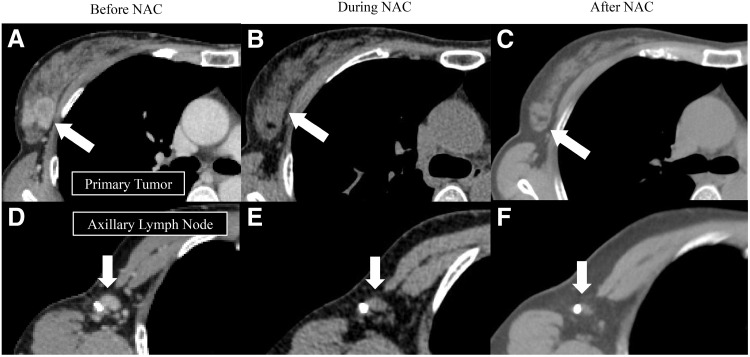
Serial CT images of the primary tumor and axillary lymph node before and after neoadjuvant therapy. (A–C) Axial CT images of the primary tumor in the right breast demonstrating gradual tumor shrinkage in response to ICI-based neoadjuvant chemotherapy. (A) Image from May of Year X (before treatment initiation), showing tumor diameter of 65 mm. (B) Image from August of Year X, after 4 cycles of pembrolizumab (200 mg every 3 weeks) combined with weekly paclitaxel (80 mg/m^2^) and carboplatin (AUC 1.5). (C) Image from December of Year X, after 4 additional cycles of pembrolizumab with epirubicin (90 mg/m^2^) and cyclophosphamide (600 mg/m^2^), showing a decrease in tumor diameter to 30 mm. (D–F) CT images of ipsilateral axillary lymph nodes. Images were captured at the same time points as those in (A–C). A progressive reduction in lymph node size was observed, indicating treatment efficacy. Notably, the lymph node was confirmed to harbor metastasis by fine-needle aspiration cytology before treatment, and a clip was placed in the node for identification. Arrows indicate the primary breast tumor in panels A–C and the ipsilateral axillary lymph node in panels D–F. AUC, area under the curve; ICI, immune checkpoint inhibitor; NAC, neoadjuvant chemotherapy
